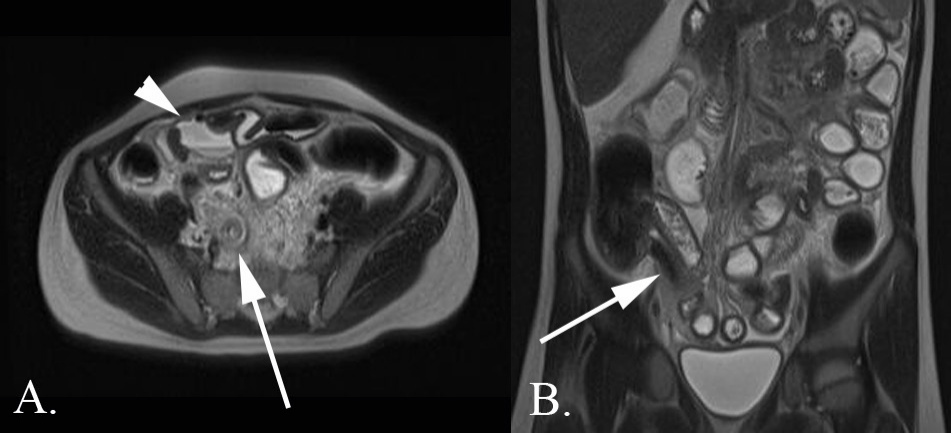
A 9-year-old male with abdominal pain who was diagnosed with complicated appendicitis. (A) Axial *T_2_* weighted MR image without fat saturation demonstrates a markedly dilated appendix (arrow) with pronounced inflammatory changes of the periappendiceal fat. There is a complex fluid collection containing a fluid*–*fluid level and a locule of free air (arrowhead). (B) Coronal *T_2_* weighted MR image without fat saturation again demonstrates a markedly dilated appendix. Small volume free fluid is noted throughout the abdomen with dilated loops of small bowel consistent with developing small bowel obstruction.
